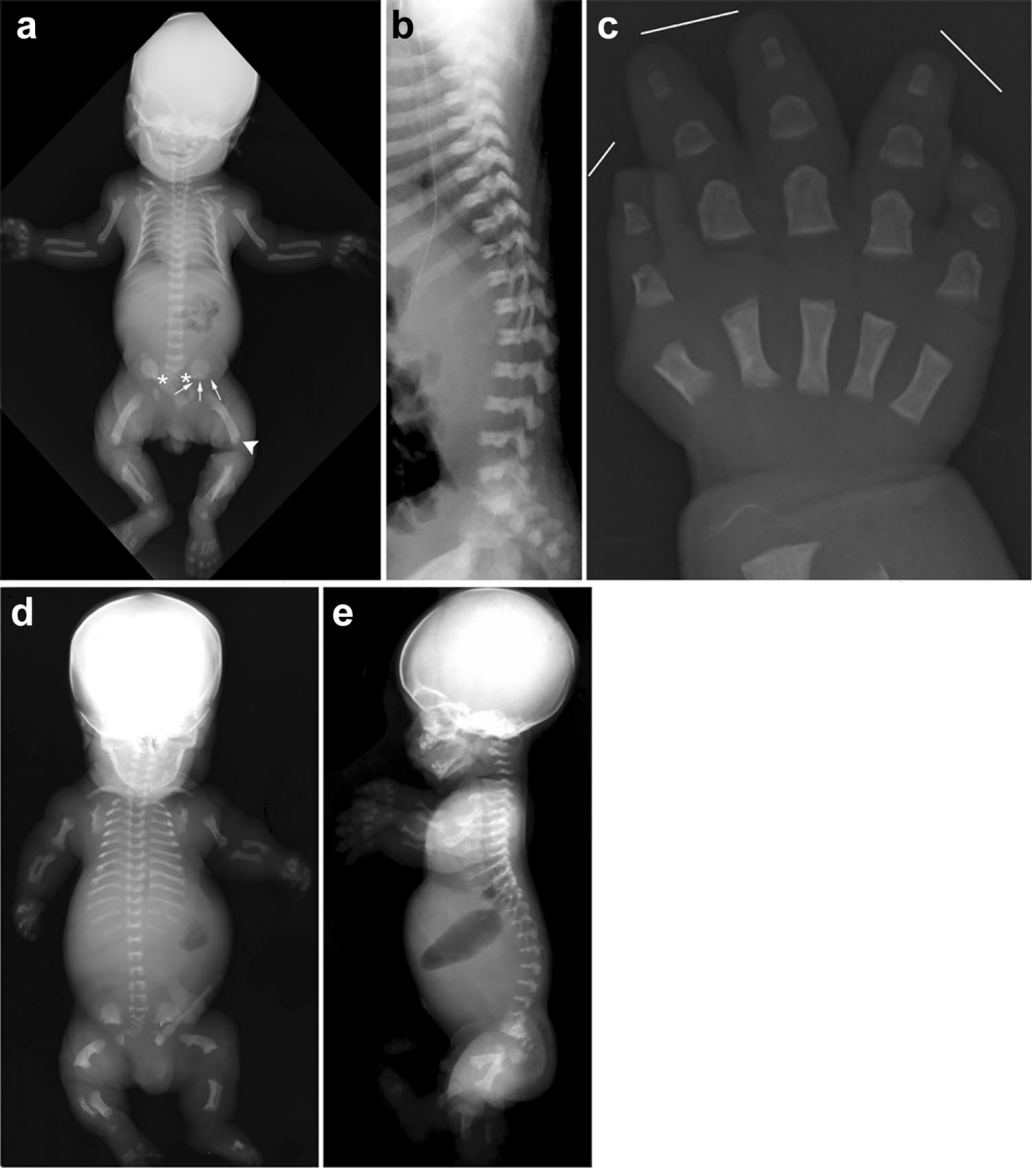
a, b A neonate with achondroplasia. a Radiographs show a disproportionately large skull, a narrow thorax, absence of normal increase in caudal interpedicle distances, small iliac bones with short sacrosciatic notches (asterisks) and trident-appearing acetabula (corniculate protrusion of the lateral, middle, and medial edge of the triradiate cartilage; arrows), ovoid lucency in the proximal femora, short and broad tubular bones associated with metaphyseal cupping, lateral slanting of the end of the distal femoral ends (arrowhead), short tibiae leading to relative elongation of the fibulae, and b “bullet-shaped” flat vertebral bodies and narrow spinal canal (on lateral view). c A frontal hand radiograph of another neonate with achondroplasia shows radial slanting of the 2nd metacarpal end and proximal phalangeal base, and a so-called “trident hand” (unable to oppose the 3rd and 4th fingers; three white lines). d, e A stillbirth with thanatophoric dysplasia. Radiographs show a disproportionately large skull, an extremely narrow thorax, severe platyspondyly (i.e., flat spine), caudally decreased interpedicle distances, small iliac bones with narrow sacrosciatic notches and trident-shaped acetabula, bowed femora (termed “French telephone receiver femora”), ovoid lucency of the proximal humeri/ femora (caused by metaphyseal cupping), metaphyseal cupping of the long bones. Note all the skeletal changes are qualitatively similar to, but quantitatively much more severe than those of achondroplasia
